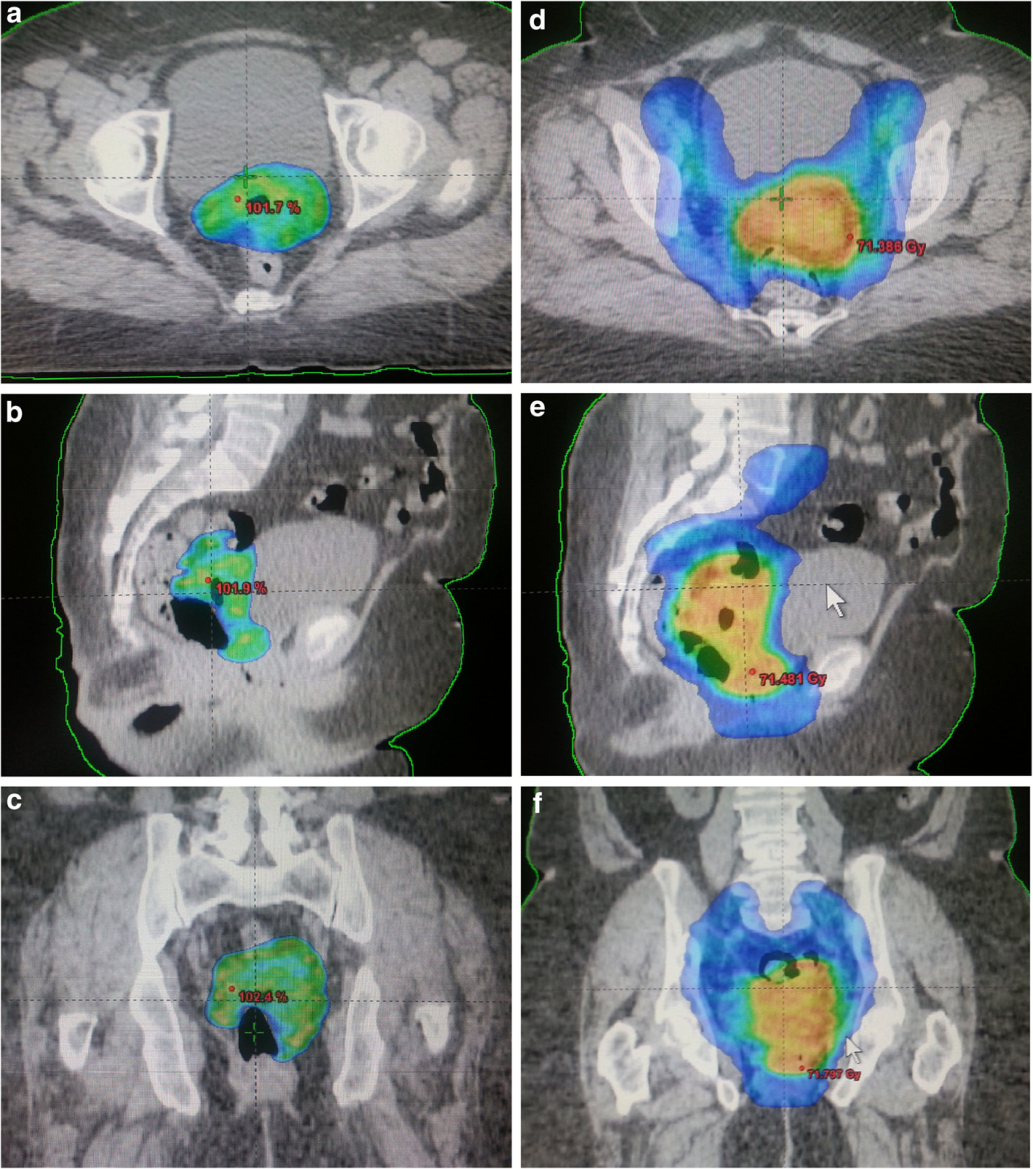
a–f Axial, sagittal, and coronal images of computed tomography scan dosimetry showed our excellent conformity for dose distribution in radiation therapy
